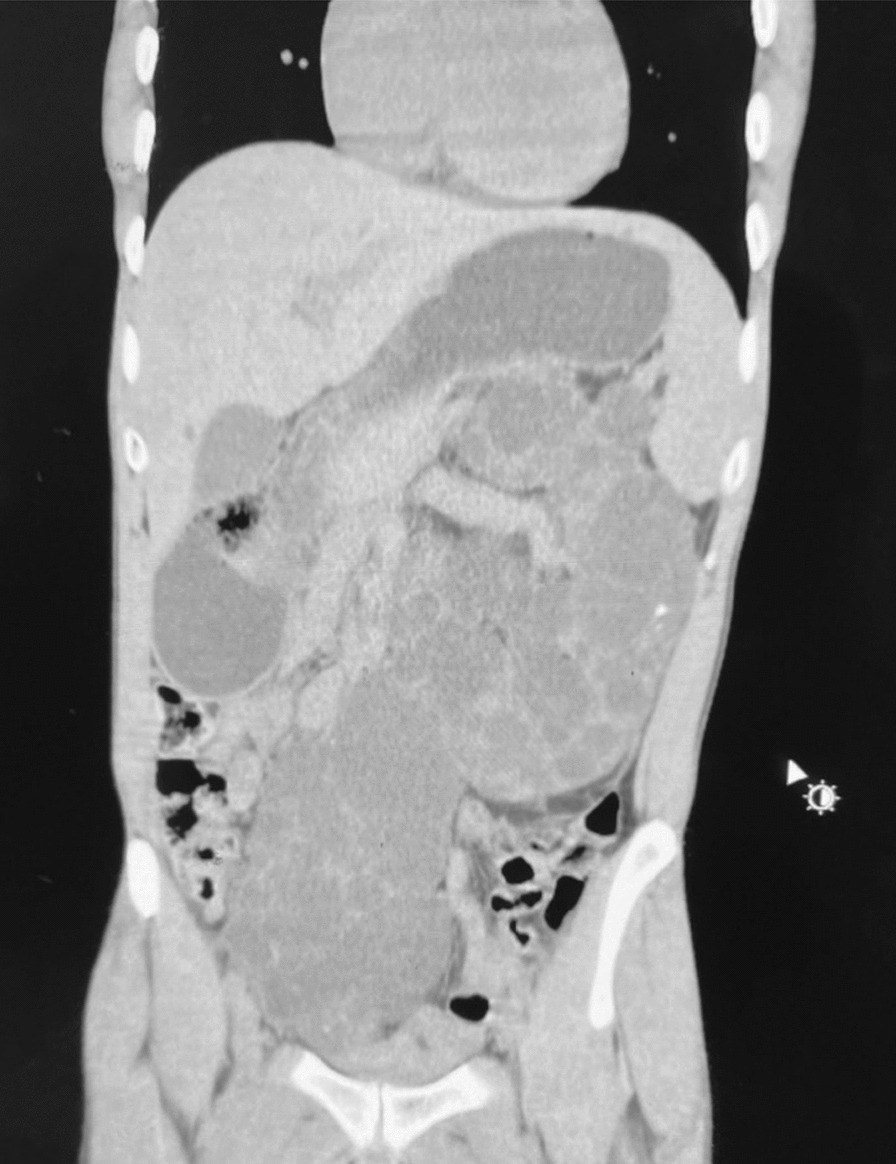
Right ectopic and left orthotopic polycystic kidney disease on CT—Scan (Coronal view)
